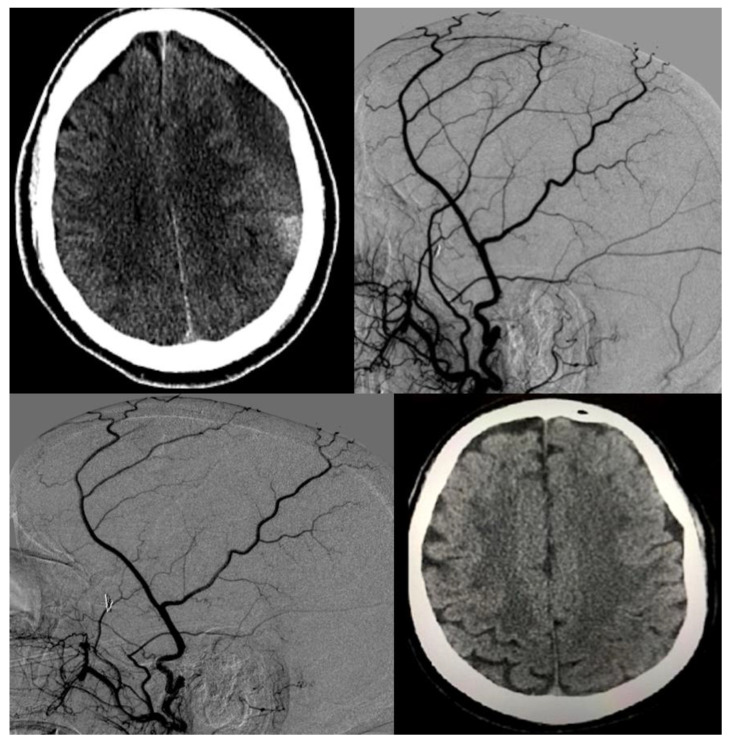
A 68-year-old man with medical history of hypertension and chronic non-valvular atrial fibrillation on coumadin had a minor motor vehicle accident with whiplash injury but no loss of consciousness. Post-trauma emergent non-contrast CT (NCCT) of the head revealed no evidence of subdural hemorrhage. Patient was asked to resume his normal activities as well as continue with anticoagulation regimen. Three weeks later while vacationing in another state, the patient developed subtle speech difficulties, gait disturbance as well as occasional memory lapses. Emergent NCCT was done which revealed bifrontal subdural hematoma (left worse than right) with acute on chronic component and midline shift. He underwent bifrontal burr hole surgeries but had a fresh re-hemorrhage a week later. The patient flew back to home state where another NCCT was prompted by clinical worsening, including coordination difficulties and word-finding difficulties. This revealed significant worsening from acute on chronic SDH as well as midline shift (top left). Patient was taken for emergent MMA embolization, and the images shows pre-embolization (top right) and post-embolization (bottom left) angiograms. Patient then had monthly clinical and radiological (NCCT) follow-ups. Anticoagulation was stopped throughout this period. Four months later, the patient had near resolution of his SDH after which antiplatelets were started. After another month, anticoagulation was started. The last control NCCT was done 3 months after resumption of anticoagulation and is shown in the image on the bottom right.
